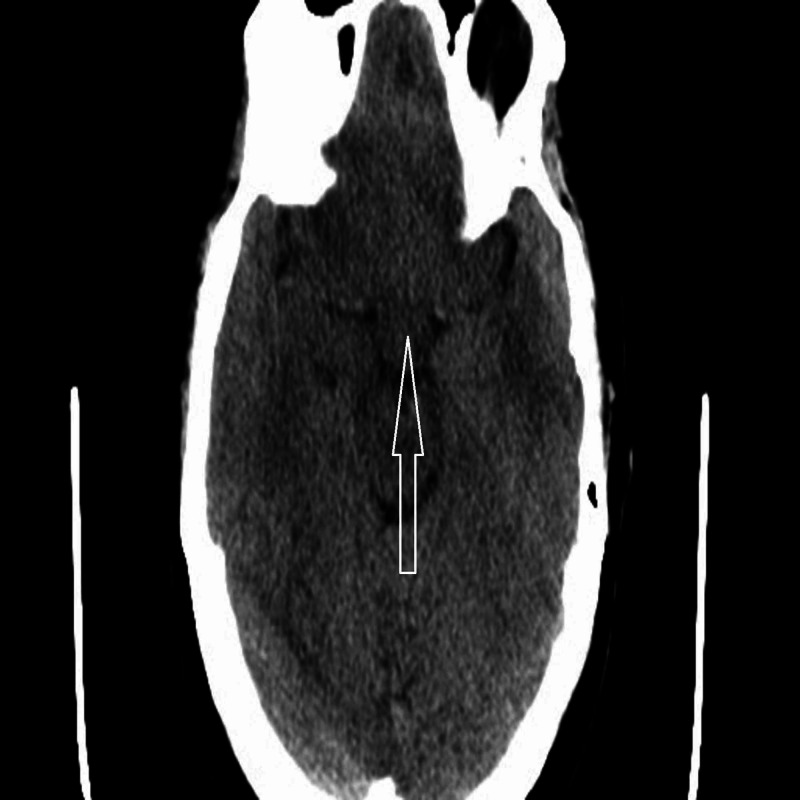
CT of the head without contrast showing a posterior suprasellar soft tissue fullness measuring 6mm in the hypothalamic region.
